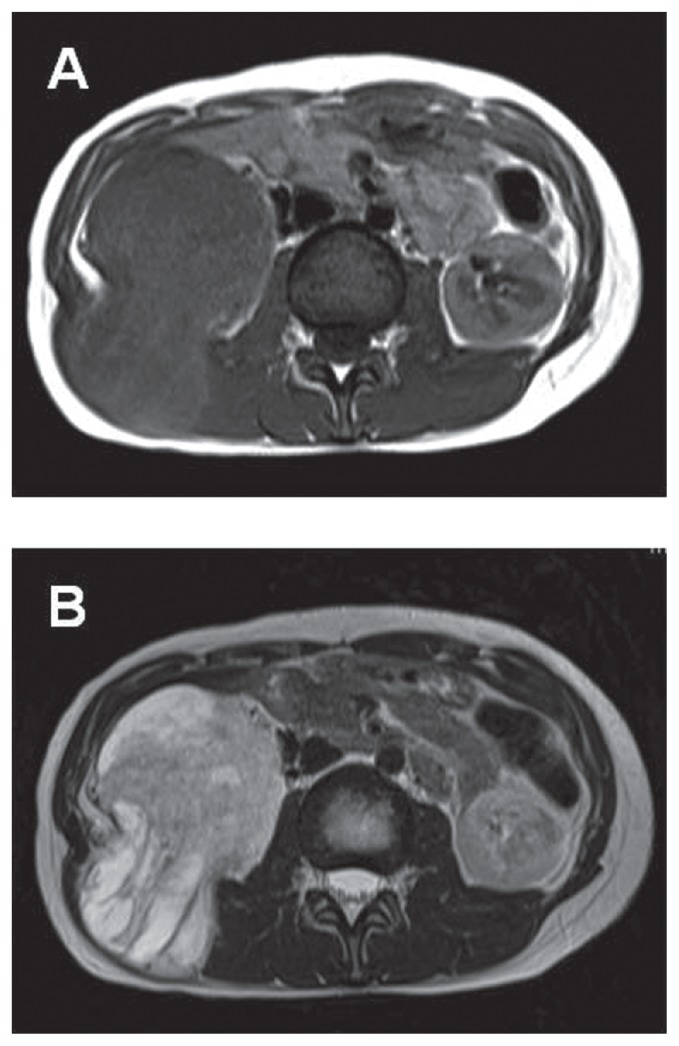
A) Magnetic resonance imaging demonstrated a tumor that had relatively smooth margin in the retoperitoneum exhibiting mainly high intensity on T1 weighting; B) T2-weighted image showed a high signal intensity tumor with low signal intensity area inside.
